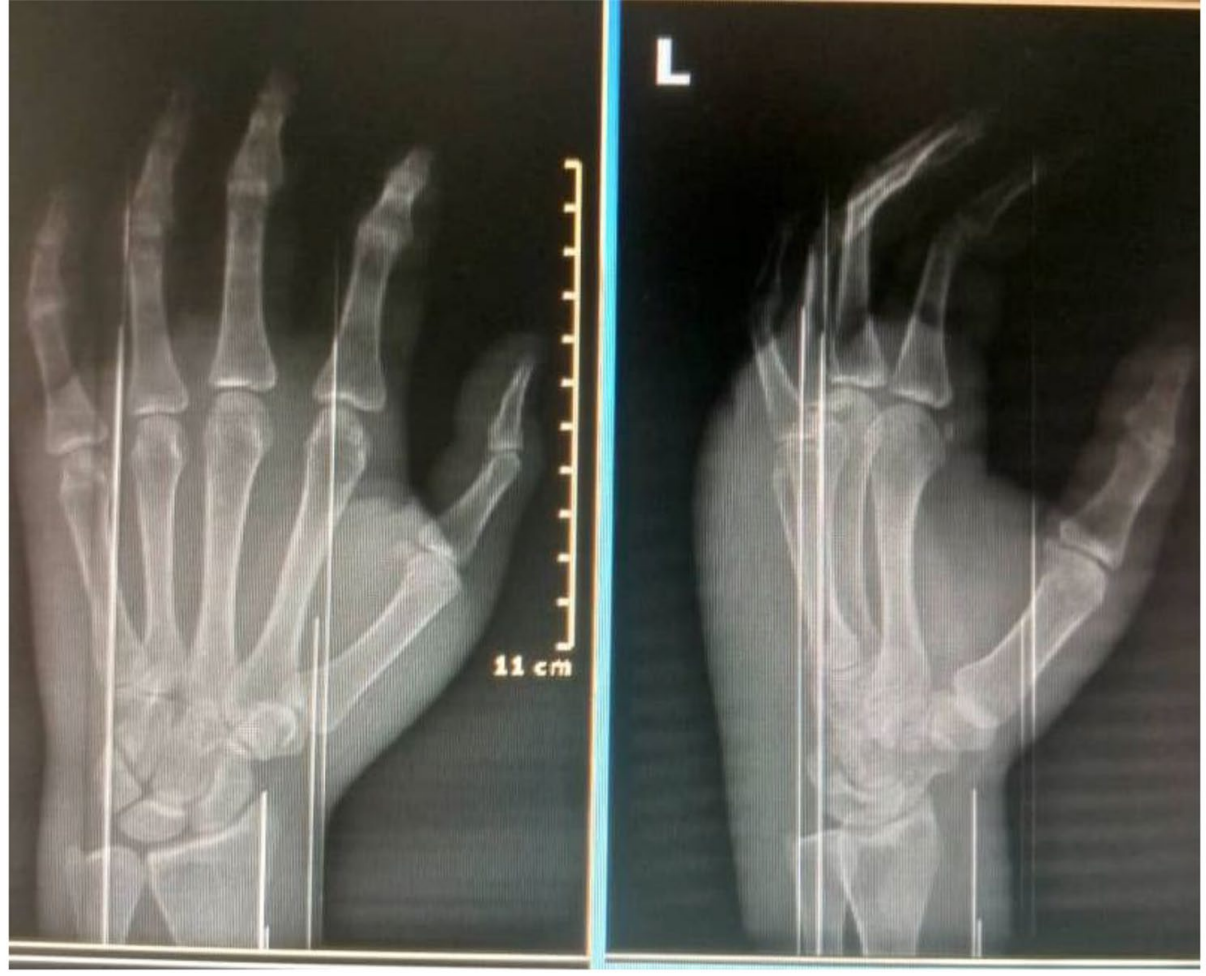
Xray image of the left hand
